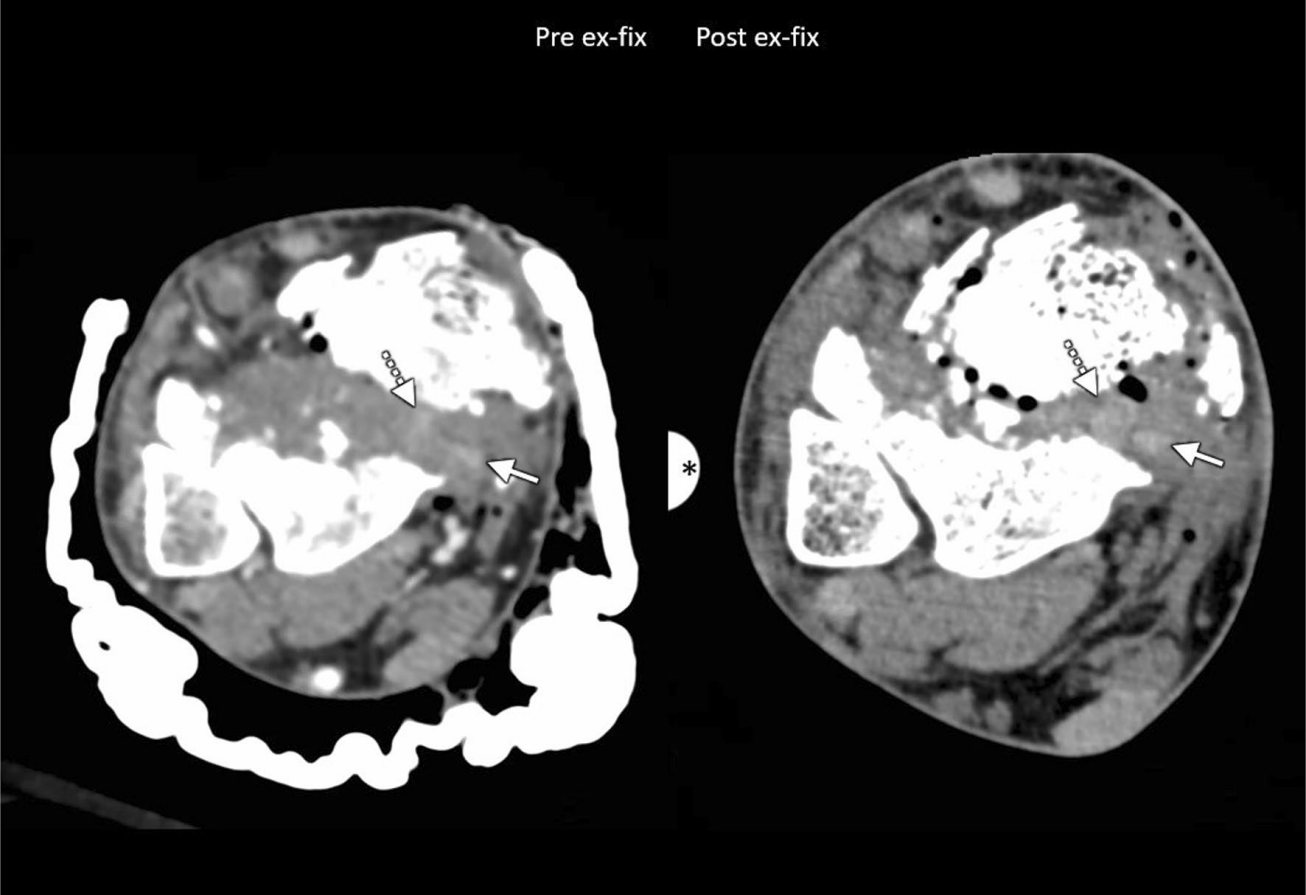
Forty-four-year-old man who fell from the US border fence, with AO/OTA 43C3.3 pilon fracture (Ruedi/Allgower type 3). Initial trauma CT angiogram (left) shows entrapment of the posterior tibial tendon (solid arrows) and flexor digitorum longus tendon (dotted arrows) within the fracture. Post-SEF CT (right) shows persistent entrapment of both tendons. External fixation device is present (asterisk)
